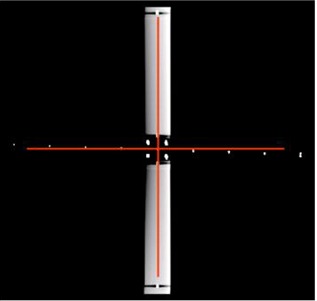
Coronal view acquired when the longitudinal arm of the jig was aligned with S line and the jig was manually moved along S line with 1.25 mm steps after acquiring each axial image. The red cross lines indicate the vertical and horizontal axes of the image. The white dots close to the horizontal axis are the CT markers on the transverse arm.
